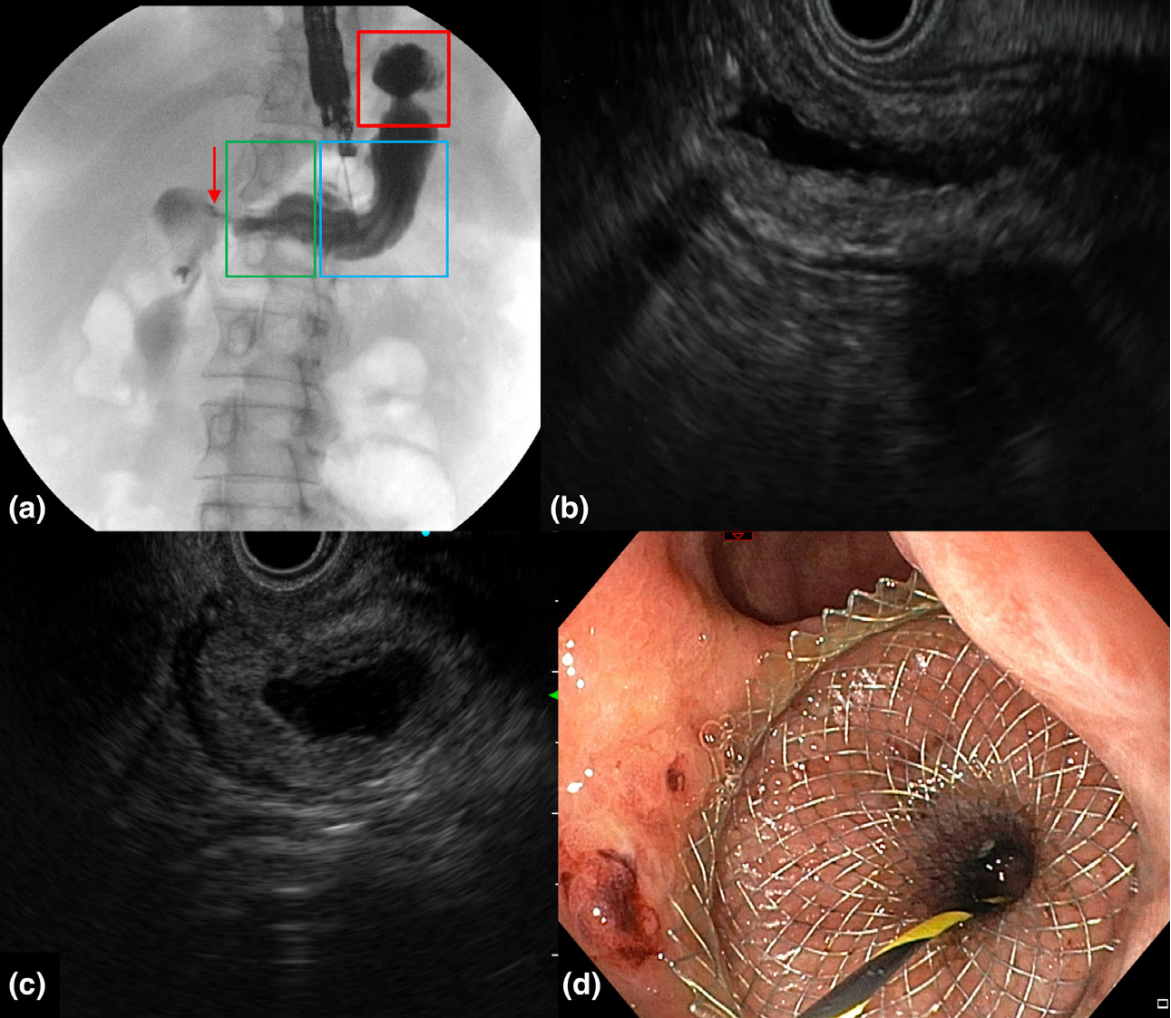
(a) Endoscopic ultrasound transgastric puncture with opacification under fluoroscopy guidance of the excluded stomach in a patient with a gastric bypass prior to deciding the better location and axis of the gastro‐gastric anastomosis. The proximal excluded stomach (red square), middle‐excluded stomach (blue square), distal‐excluded stomach (green square), and pylorus (arrow) can be identified. (b) Endoscopic ultrasound image of the middle‐excluded stomach, usually less dilated and with a larger horizontal size compared to the (c) proximal‐excluded gastric cavity. (d) Endoscopic image of a lumen‐apposing metal stent deployed in transgastric position a few centimeters above the surgical gastro‐jejunal anastomosis.
